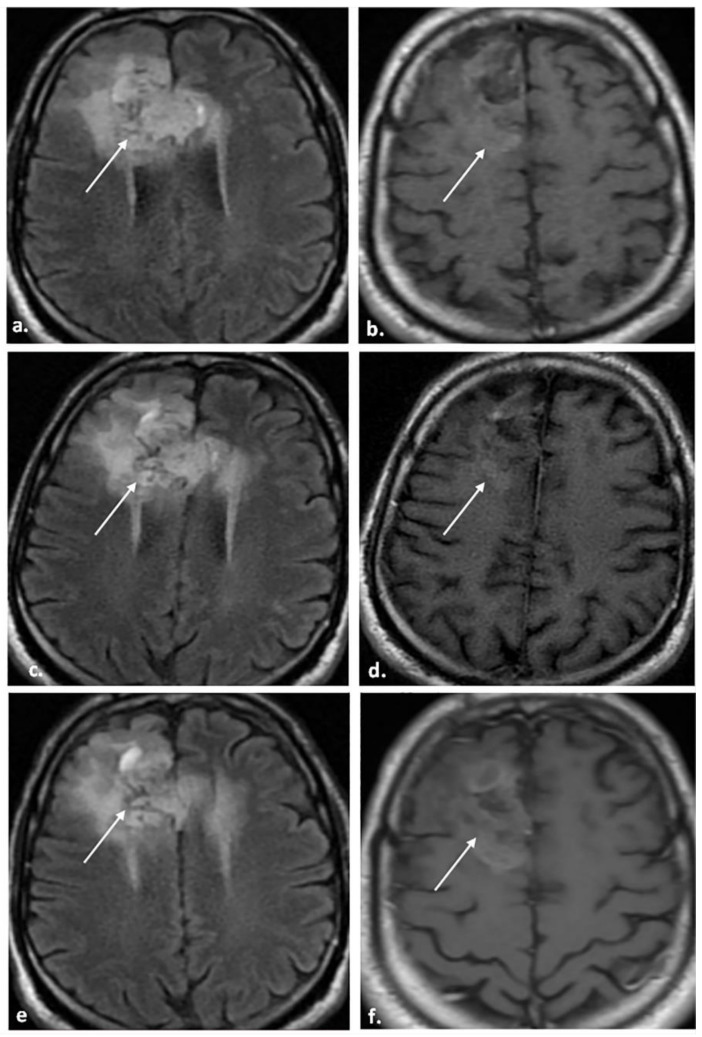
A 50-year-old male patient underwent total resection of HGG. The patient did not receive antiangiogenic therapy. (a,b) MRI was performed 4 months after the completion of radiotherapy. (a) The axial FLAIR image shows a right frontal high signal intensity area at the operative bed (arrow) extending to the genu of the corpus callosum to the opposite side with a mild positive mass effect on the related cortical sulci. (b) The axial post-contrast T1WI image shows no enhancement (arrow). (c,d) A follow-up MRI was performed 8 months after the completion of radiotherapy; the patient was clinically stable. (c) The axial FLAIR image shows a stable FLAIR component and mass effect (arrow). (d) The axial post-contrast T1WI image shows a stable enhancement pattern (arrow). The patient was categorized as BT-RADS-2. (e,f) A follow-up MRI was performed 12 months after the completion of radiotherapy; the patient was clinically stable. (e) The axial FLAIR image shows a stable FLAIR component and no mass effect (arrow). (f) The axial post-contrast T1WI image shows a stable enhancement pattern (arrow). The multidisciplinary brain tumor team considered the lesion to be non-progressive.
